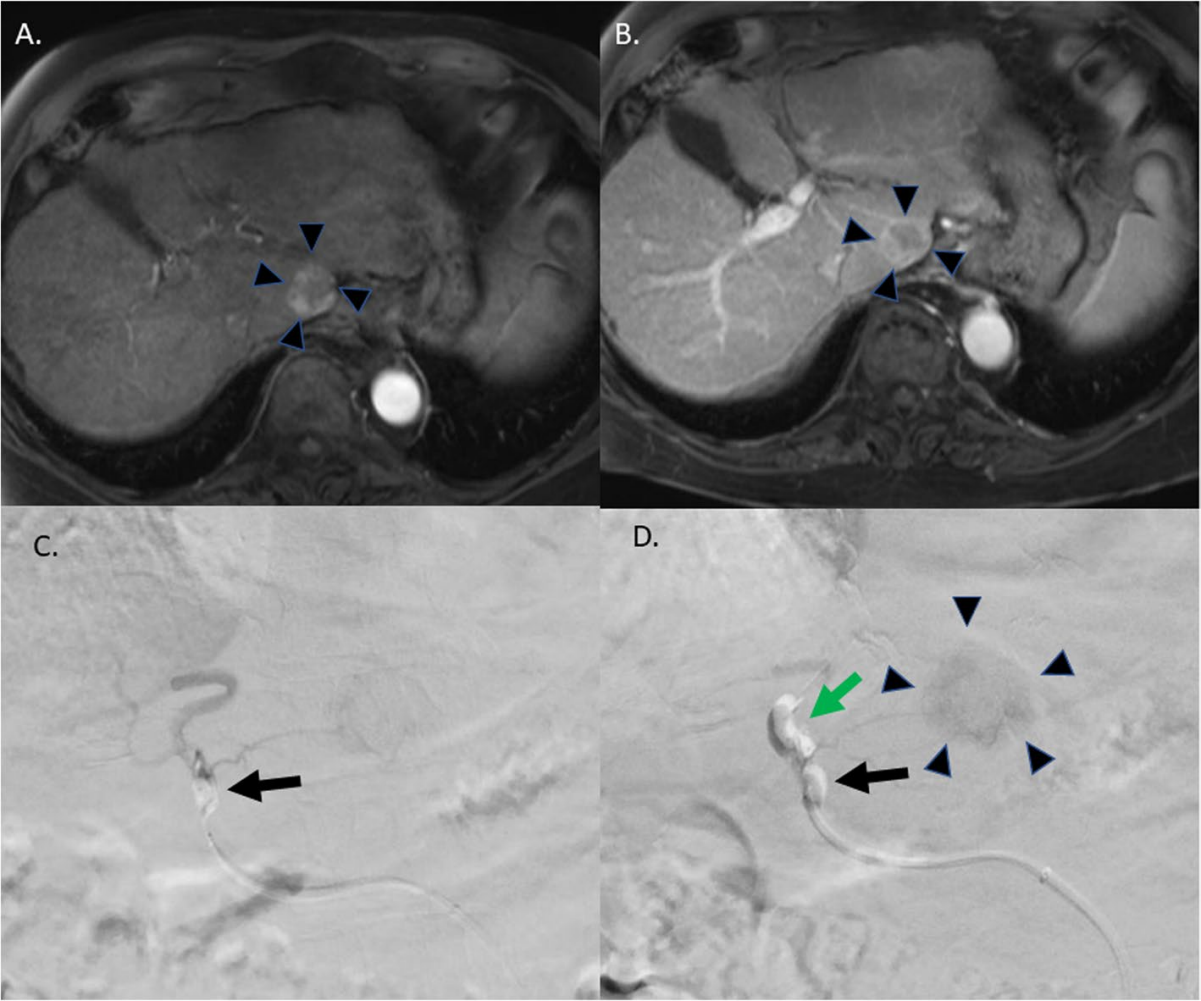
a-d Treatment of a OPTN5B caudate lesion. a Surveillance MRI in this patient with cirrhosis demonstrated an arterially enhancing lesion measuring 2.3 cm centered in segment 1(black arrow heads) with b early washout and pseudocapsule formation deeming it OPTN5b and was referred for Y-90 radioembolization. His Pre-SIRT and SIRT studies demonstrated the caudate lobe artery arising from the proximal left hepatic artery with single tumor blush but with substantial adjacent supply to segments II/III and IV. Given desire to reduce risk of hepatic parenchymal embolization due to comorbidities of cirrhosis and portal hypertension, the double balloon technique was used. A 2.1 French Sniper catheter was positioned beyond the caudate artery. c A second 2.1 French angled Sniper catheter was placed and positioned at the proximal left hepatic artery proximal to the caudate artery (black arrow). d Both balloons were inflated with dilute contrast per manufacturer protocol (green and black arrows). Digital subtraction angiography from the proximal catheter demonstrated selective flow of contrast into caudate artery with avid enhancement of target lesion (black arrow heads), and minimal, sluggish flow beyond the second/distal Sniper balloon, so Y-90 Theraspheres were administered
